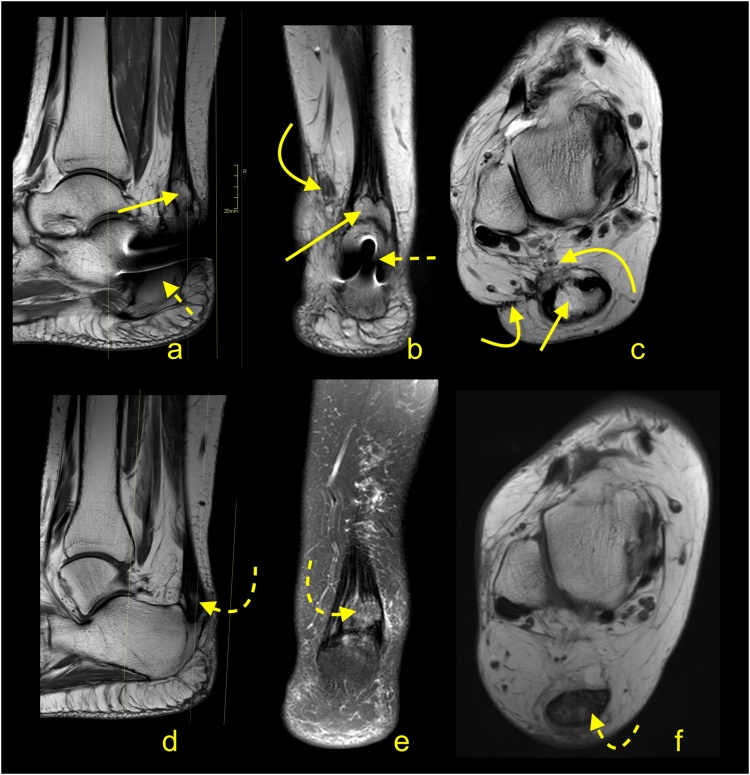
Recurrence of Haglund’s syndrome after surgery. A 71-year-old patient presented with pain and thickening of the Achilles tendon insertion about 1 year after surgery. Postoperative MRI (a–c) showed exostosis (straight arrow) in the Achilles tendon insertion, which is slightly bigger than the preoperative exostosis (curved dashed arrow) revealed on preoperative MRI (d-f). Fibrotic postoperative changes (curved arrow) are visible in Kager's fat pad. Screws in the calcaneus (dashed arrow). A- PD- weighted, b and c- T2- weighted, d- PD- weighted, e- T2- weighted with fat suppression, f- T1- weighted.
